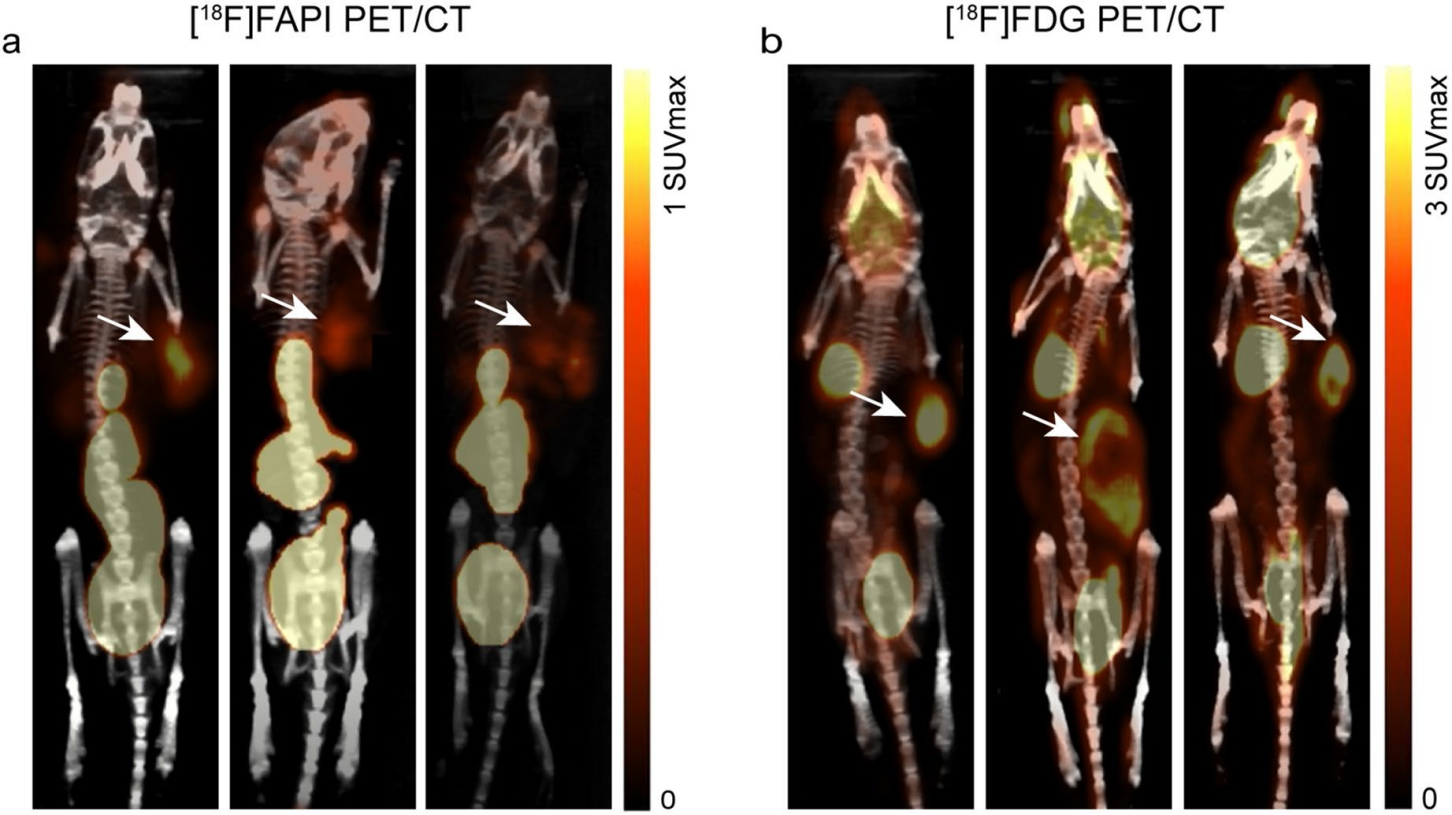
Comparison of uptake rates between [^18^F]FAPI-04 (1 h after injection) and [^18^F]FDG (1 h after injection) PET imaging in LLC xenograft model. (Arrows indicate tumor xenograft)
